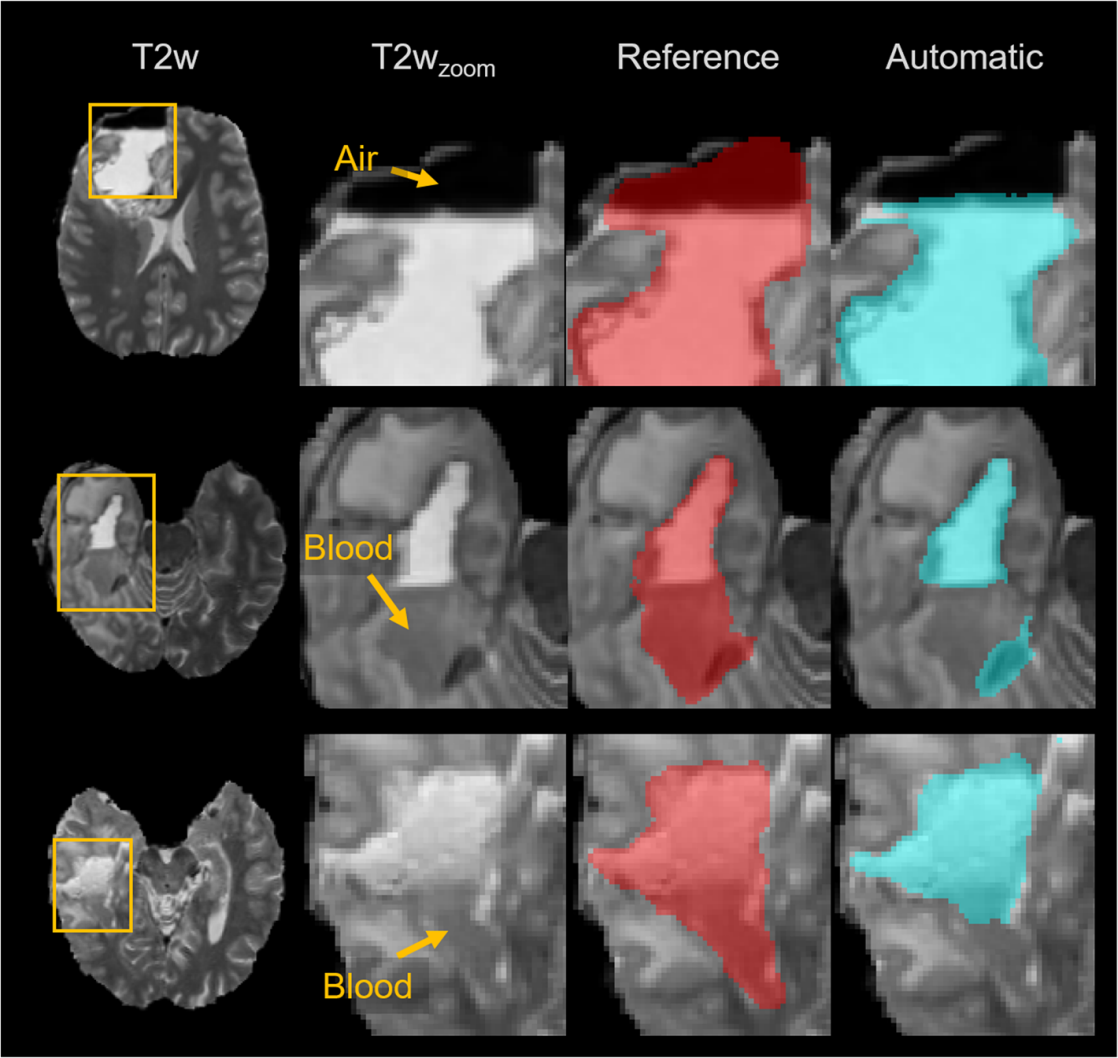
Segmentation errors introduced by air pockets and blood products. The rows indicate erroneous cases and the columns show T2-weighted images, zoomed T2-weighted images, expert consensus segmentation (reference) overlays and automatic segmentation overlays
